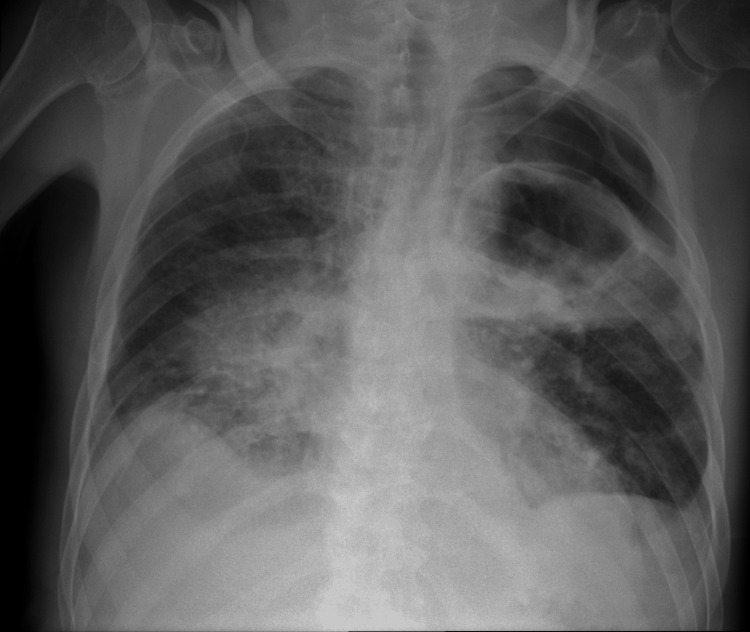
Initial chest X-ray demonstrating multifocal pulmonary involvement There are prominent right perihilar airspace opacities. Notably, a large, thick-walled cavitary lesion is visualized in the left lung apex.
